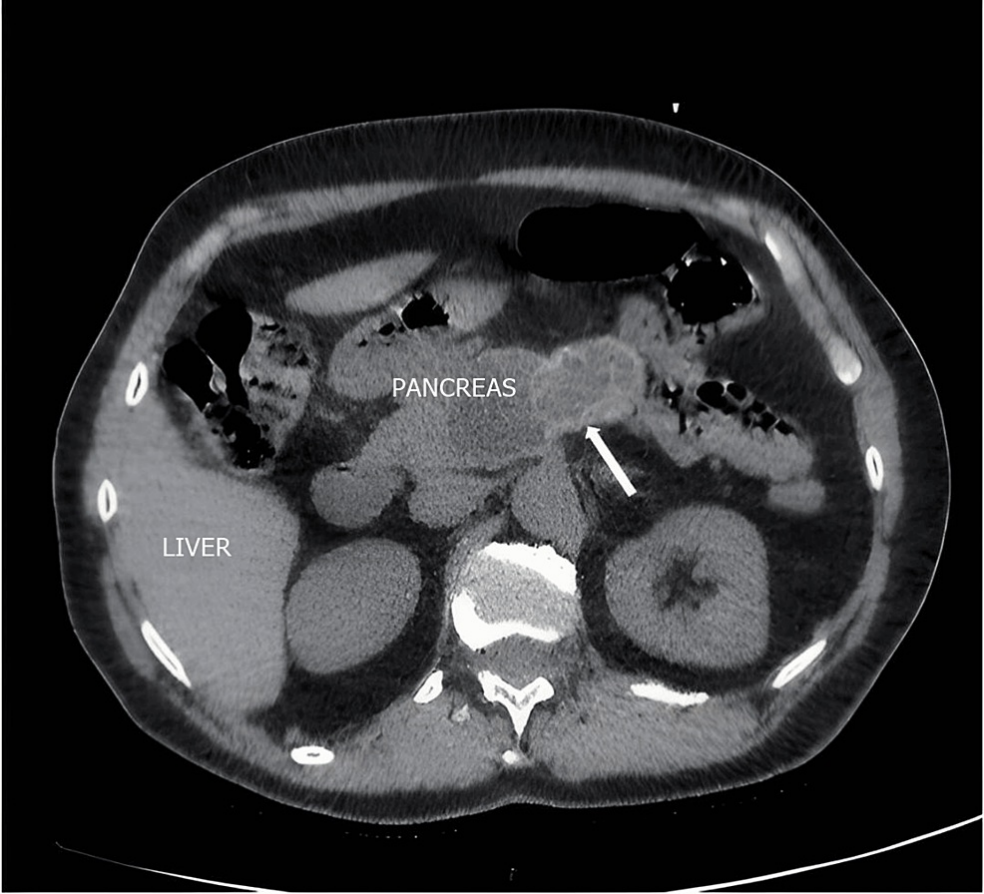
Pancreatic hydatid cyst (indicated by a white arrow): CT image in the axial section (personal case study).
